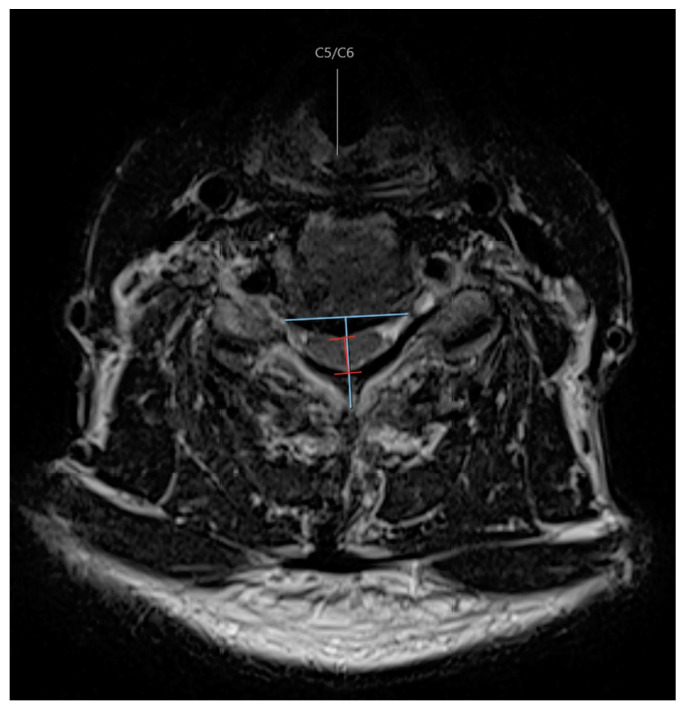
Axial T2-weighted MRI demonstrating radiographic measurements to determine the side of compression and canal diameter.
